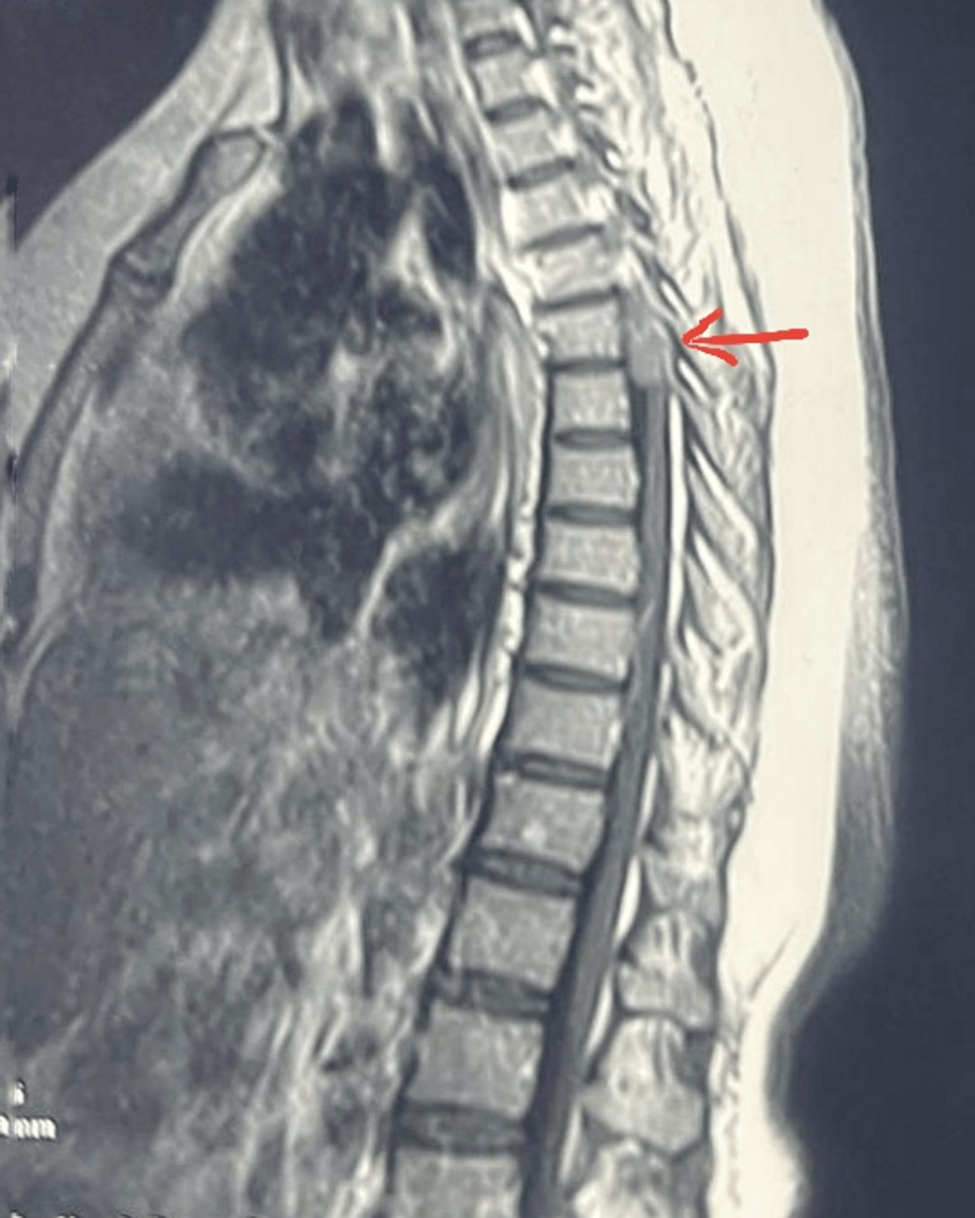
Post-gadolinium contrast T2 diffusion-weighted MRI film showing hyper intense space-occupying lesion at the level of T4-T6 vertebrae
